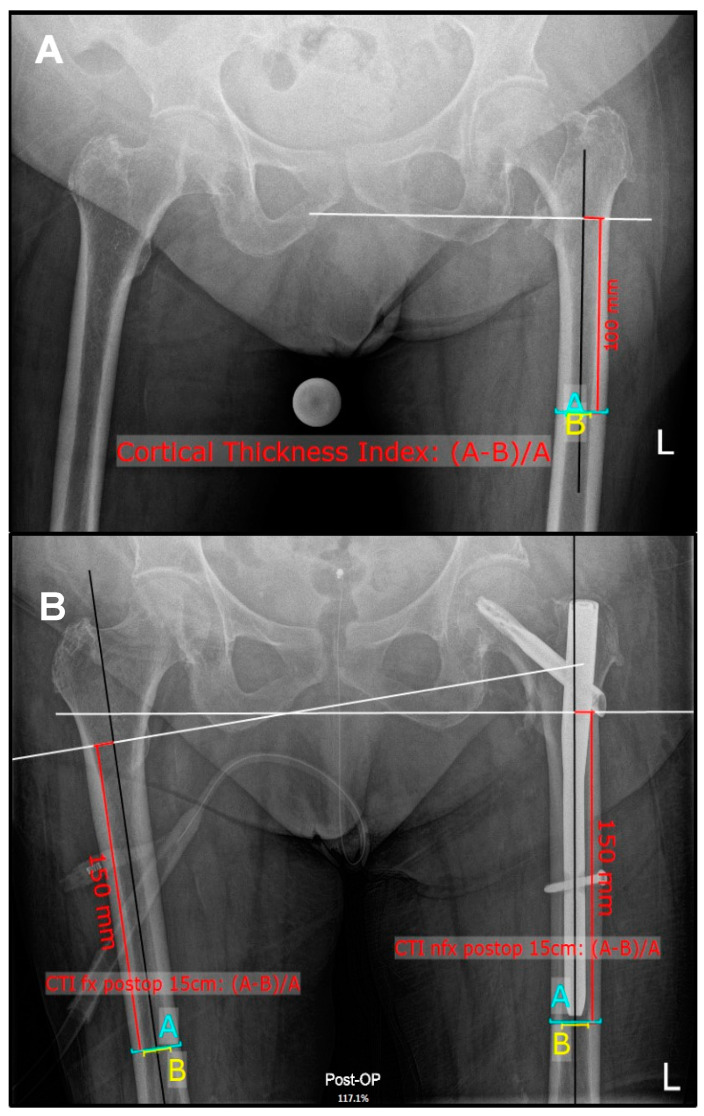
Anteroposterior X-ray of the pelvis: measurement of CTI at ten centimeters below the mid lesser trochanteric line in the left, non-fractured femur (A) and 15 cm bilaterally, after implantation of an endomedullary nail (B).
